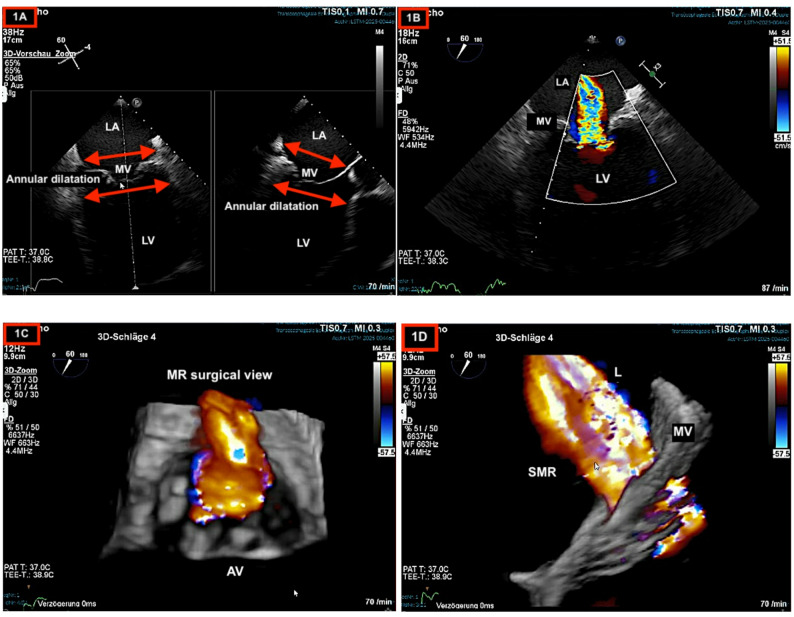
Transesophageal echocardiography demonstrating (A) the presence of atrioventricular ring dilatation, (B) sever central mitral regurgitation, (C) three-dimensional mitral regurgitation in surgical view, (D) three-dimensional mitral regurgitation in lateral view. *LA* Left atrium, *MV *Mitral valve, *LV* Left ventricle, *AV* Aortic valve, *SMR* Secondary mitral regurgitation
